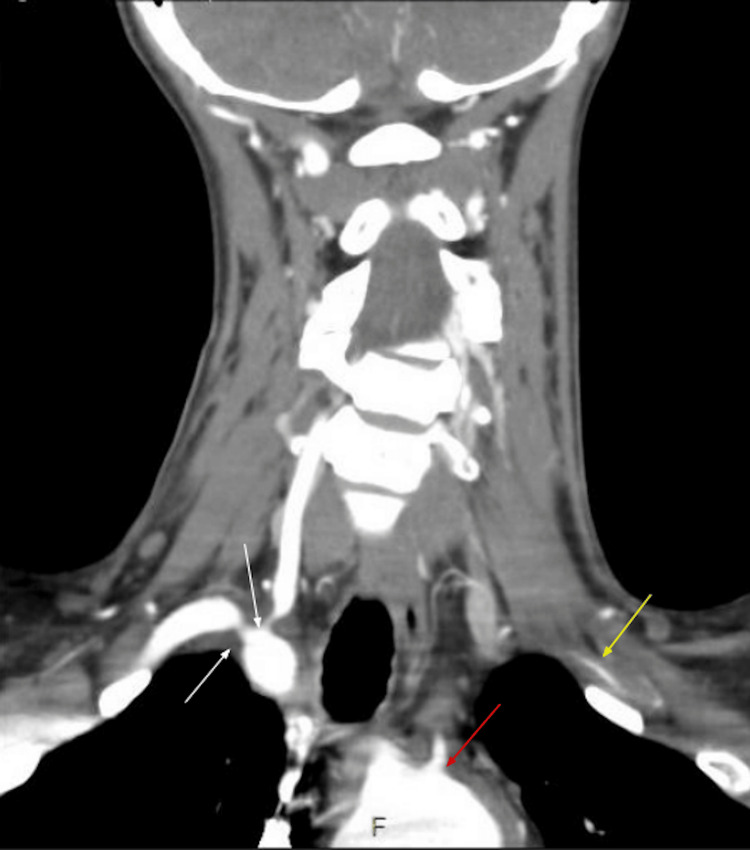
CT angiogram TAVR protocol showing multiple arterial narrowing The white arrows point to the right subclavian artery and original right vertebral artery on the right. The yellow arrow shows the left subclavian artery. The red arrow points towards the aortic arch. CT: computed tomography; TAVR: transcatheter aortic valve replacement
